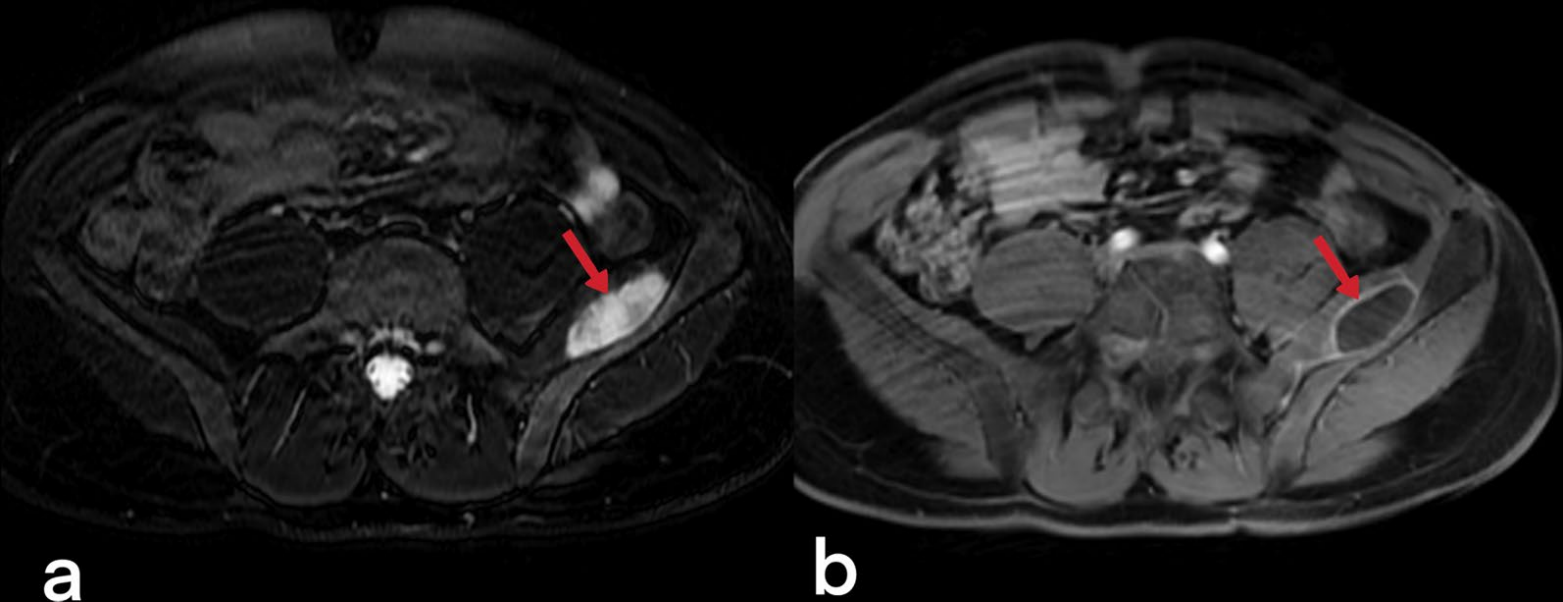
T2 weighted (a) and post-contrast T1 weighted MR images of a 35-year-old male demonstrate abscess formation with peripheral enhancement in the left iliac muscle (b)
